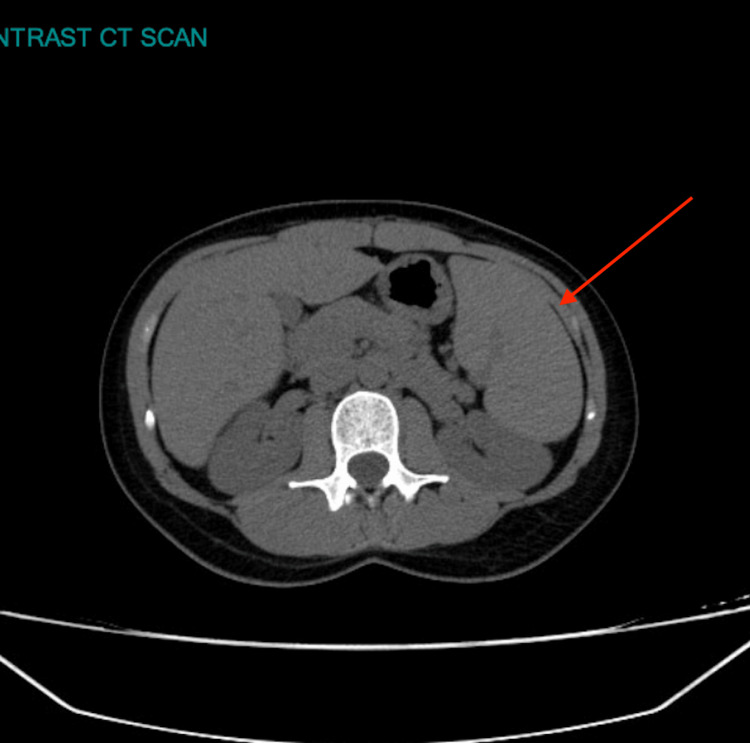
Axial CT scan of the abdomen The red arrow is pointing at the splenomegaly.
